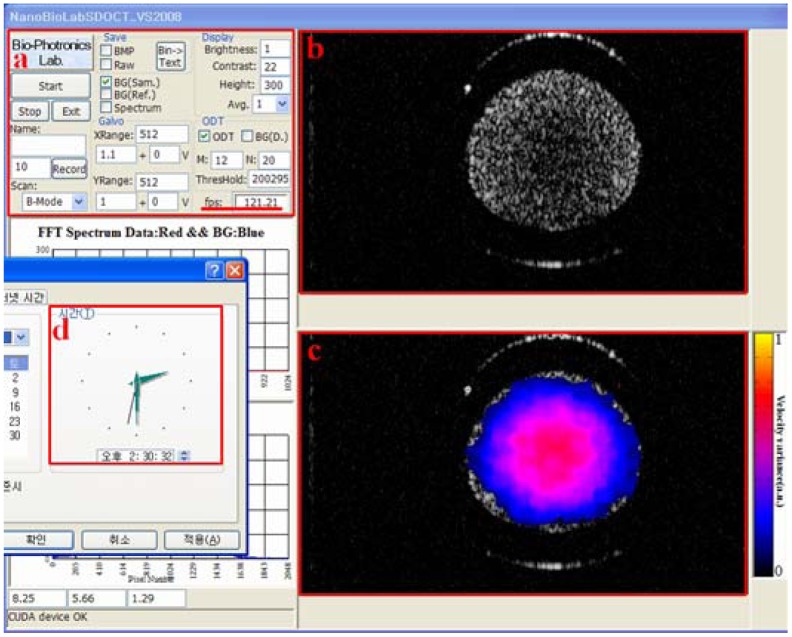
Captured movie of experiment for a capillary tube ODT. Doppler angle: 80°. Averaging window size: 12 + 20 (*M* + *N*). Displaying image size: 512 W × 300 H pixel. (a) System setup. Red line: actual frame rate; (b) OCT image; (c) ODT image; (d) The watch for time comparison.
